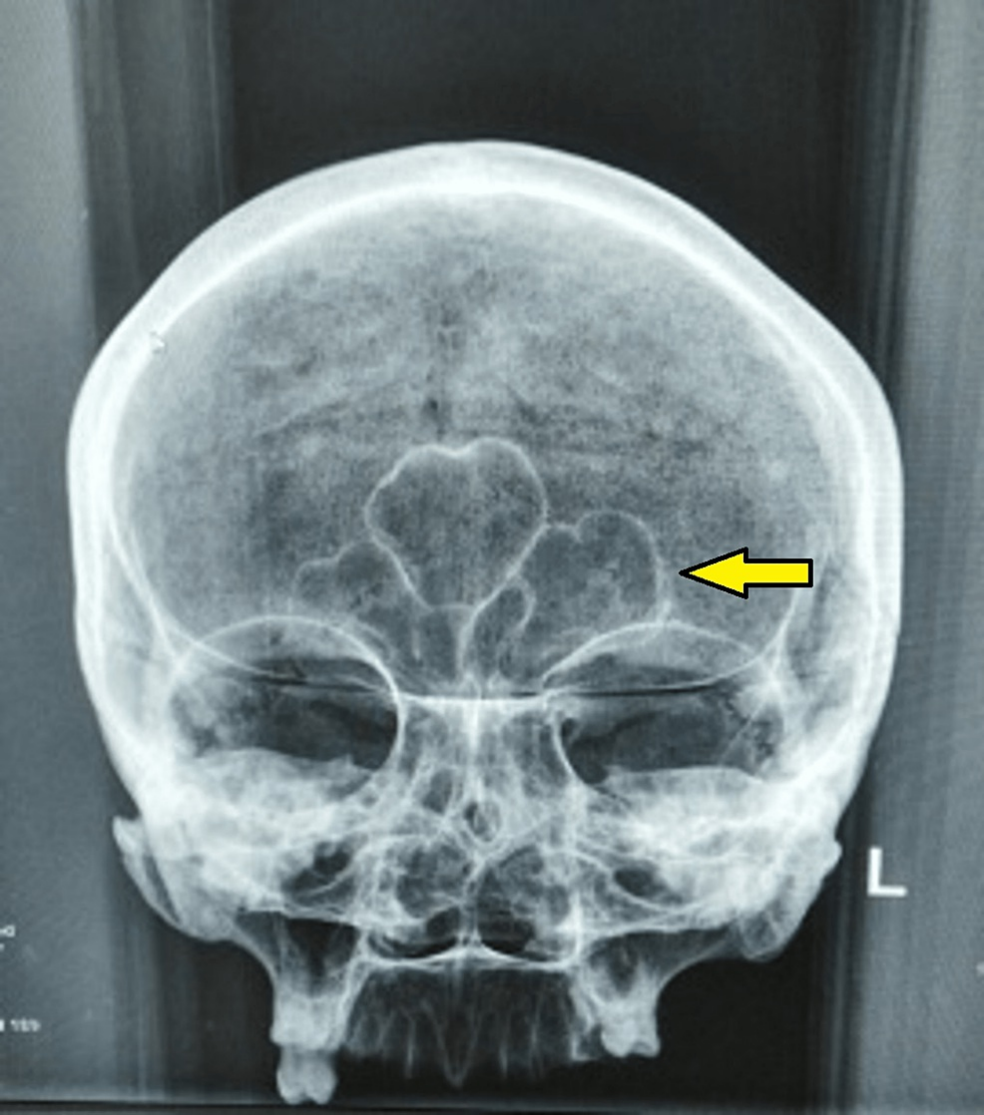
The plane X-ray skull of 50 years + female in AP shows frontal air sinuses marked by a yellow arrow.
